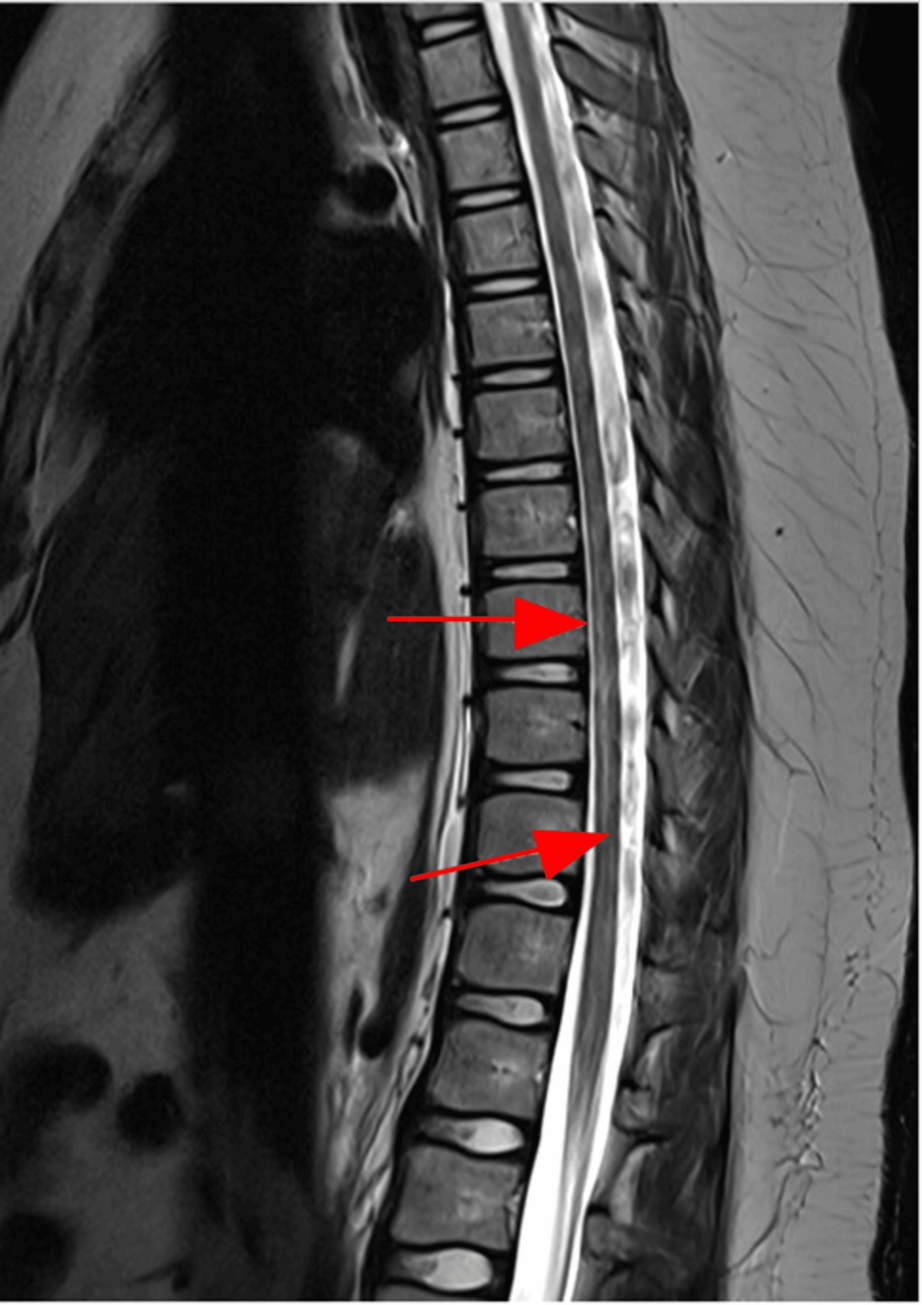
Sagittal T2-weighted MRI of the thoracic spine showing ventral-dominant cord signal abnormality from T8 to T9 through T11 to T12, indicated by red arrows
